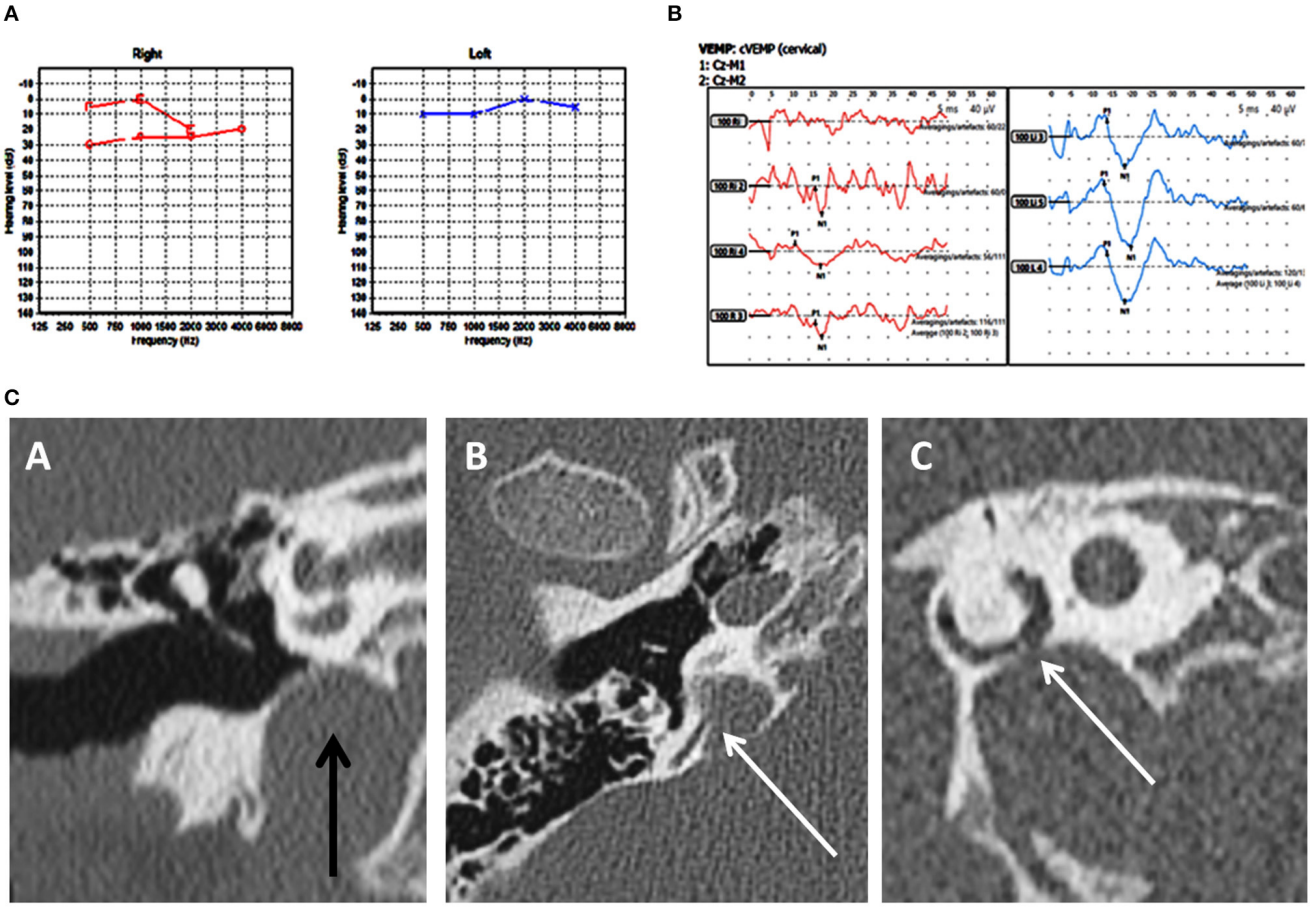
Case number 2—Unilateral right posterior semicircular dehiscence. (A), Pure tone audiometry showing mild conductive low frequency hearing loss on the right. (B), Clinically significant cVEMP amplitude asymmetry with the right weaker than the left. This child perceived significant balance issues but his vestibular tests including the vHIT was normal. (C)—CT scan images of the right petrous temporal bone in the coronal plane (A) demonstrates a high riding jugular bulb. The axial image (B) the sagittal oblique reconstruction parallel to the posterior semi-circular canal (C) demonstrate dehiscence of the posterior semi-circular canal at its junction with the jugular bulb.
